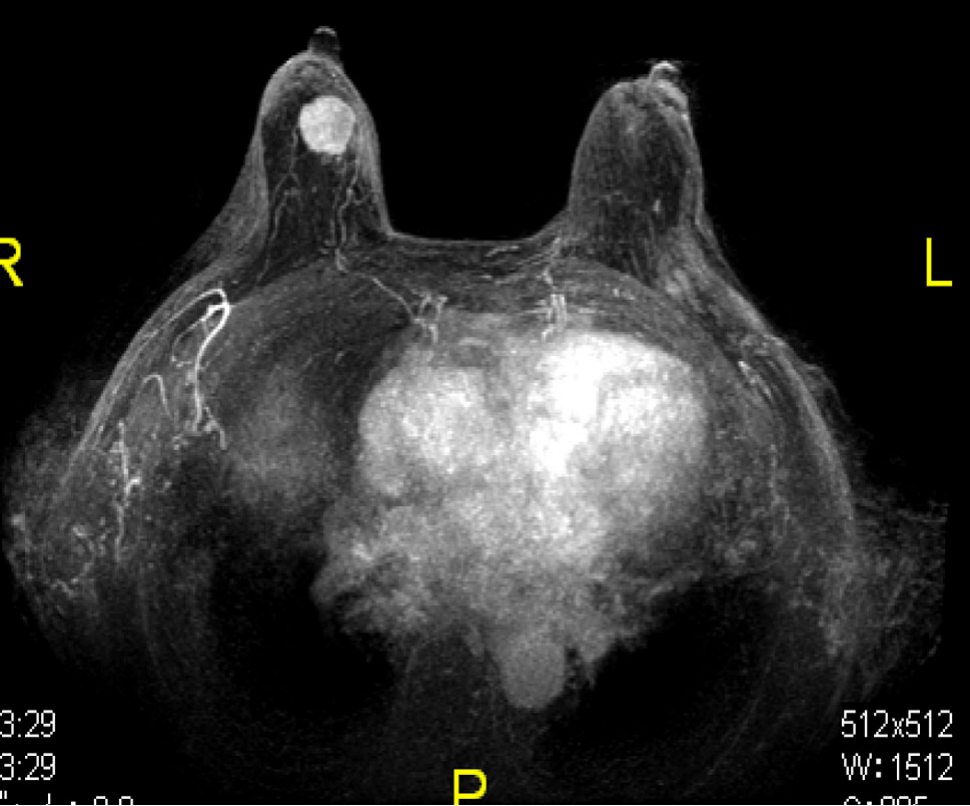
Magnetic resonance imaging findings. A 3 × 2 × 2 cm mass in the *upper right* breast showed a malignant imaging pattern on dynamic study
